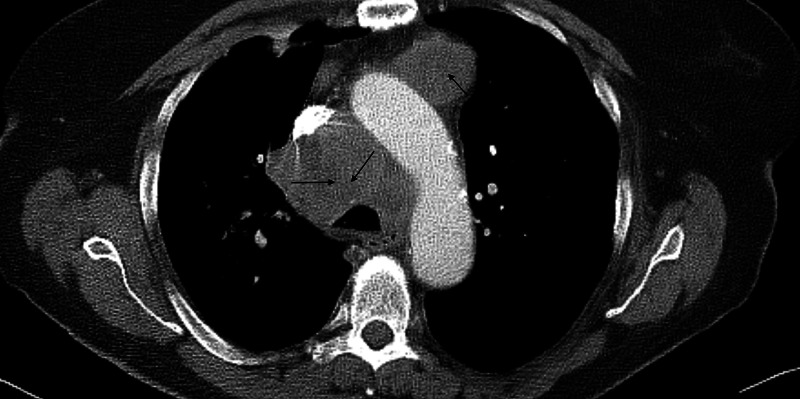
Axial CT scan chest The single arrow shows enlarged anterior mediastinal lymph nodes, and the double arrows indicate enlarged right paratracheal lymph nodes, respectively. CT: computed tomography
